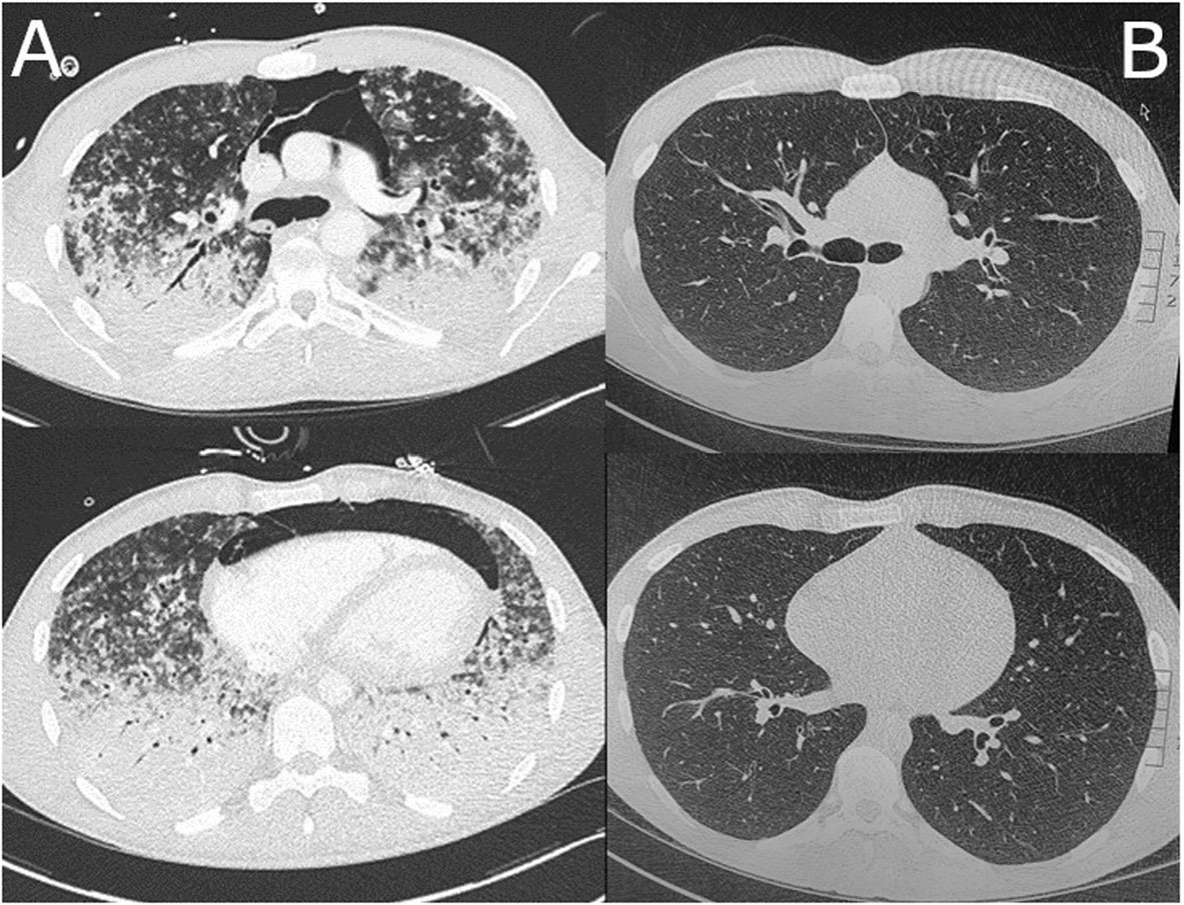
High-resolution CT images on day 1 of ECMO (a) and 9 months post-discharge (b)
